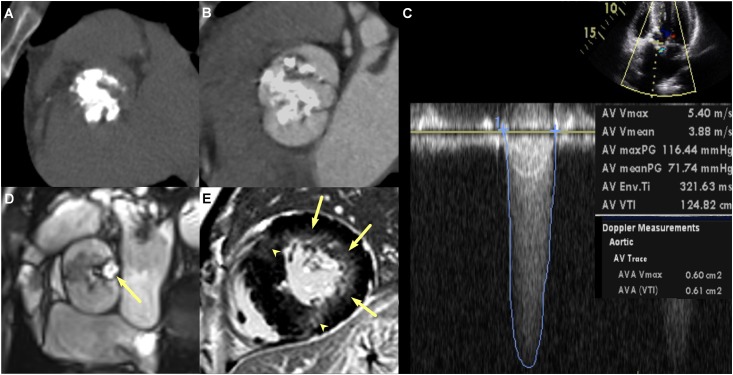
Multi-modalities images of a 45-year old man with severe aortic stenosis (AS), mild aortic regurgitation, and myocardial fibrosis (MF). a and b Cardiac computed tomography images demonstrate calcified AS (aortic valve calcification grade 4, aortic valve calcium score 7650, and aortic valve area 0.83cm^2^) and bicuspid aortic valve without raphe. c Transthoracic echocardiography image demonstrate severe AS (aortic valve area 0.61cm^2^), peak aortic velocity 5.4m/sec, and mean pressure gradient 71.7mmHg. d and e Cardiac magnetic resonance images demonstrated severe AS (aortic valve area 0.71cm^2^, arrow in d), left ventricular (LV) hypertrophy, LV end-diastolic volume index 84ml/m2, LV end-diastolic mass 268g, LV ejection fraction of 56%, presence of MF appearing delayed hyperenhancement in the subendocardial region of the lateral wall (arrows, e) and in the transmural region of the anterior and inferior wall of the mid-ventricular myocardium (arrowheads, e).
